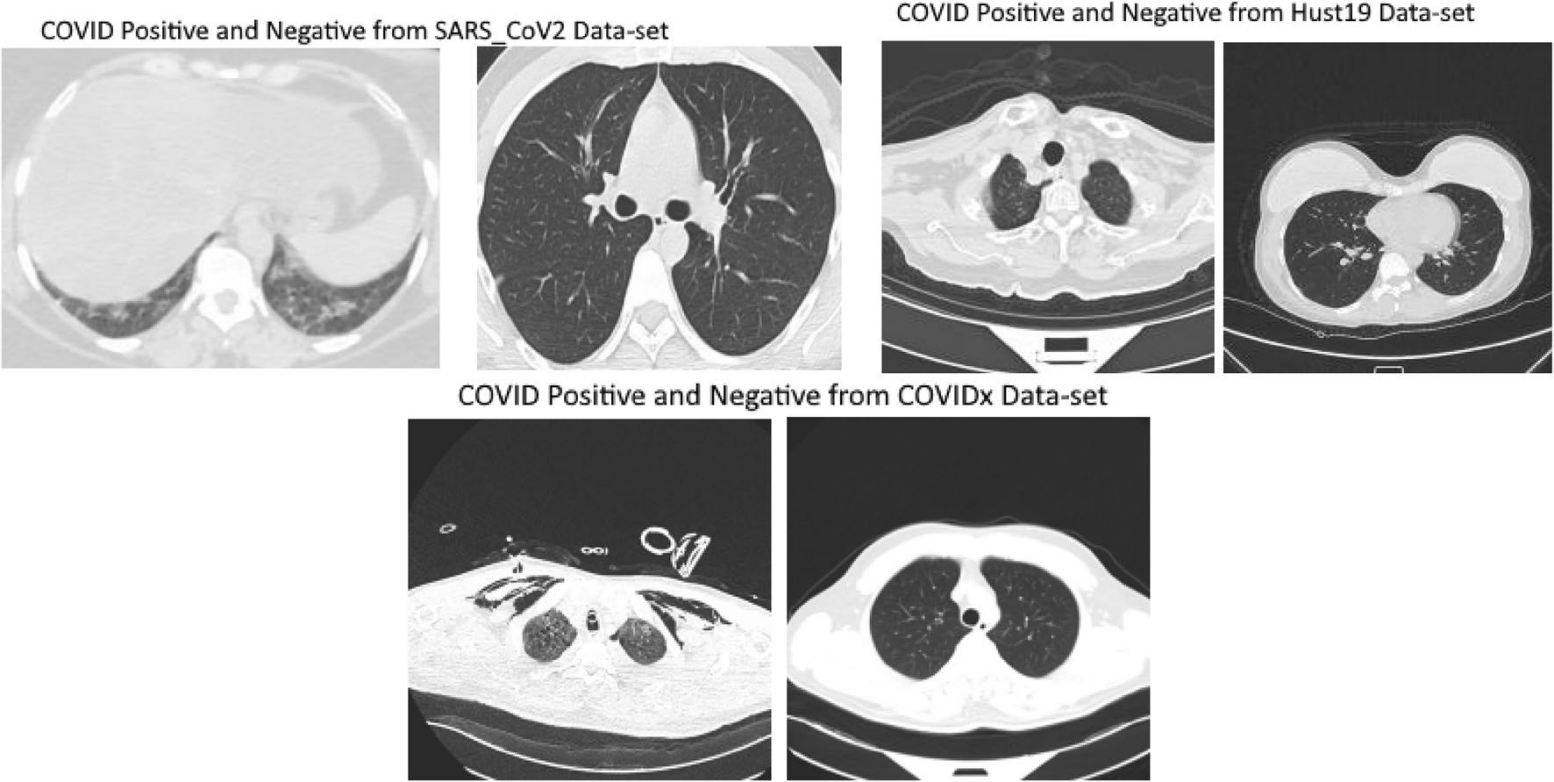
(1): examples of 3D CT scan images that are COVID positive (Left) and (2) non-COVID-19 (Right) from SARS_CoV2 Data-set. (3)Examples of 3D CT scan images that are COVID positive (Left) and (4) non-COVID-19 (Right) from Hust19 Data-set. (5) Examples of 3D CT scan images that are COVID positive (Left) and (6) non-COVID-19 (Right) from COVIDx Data-set.
